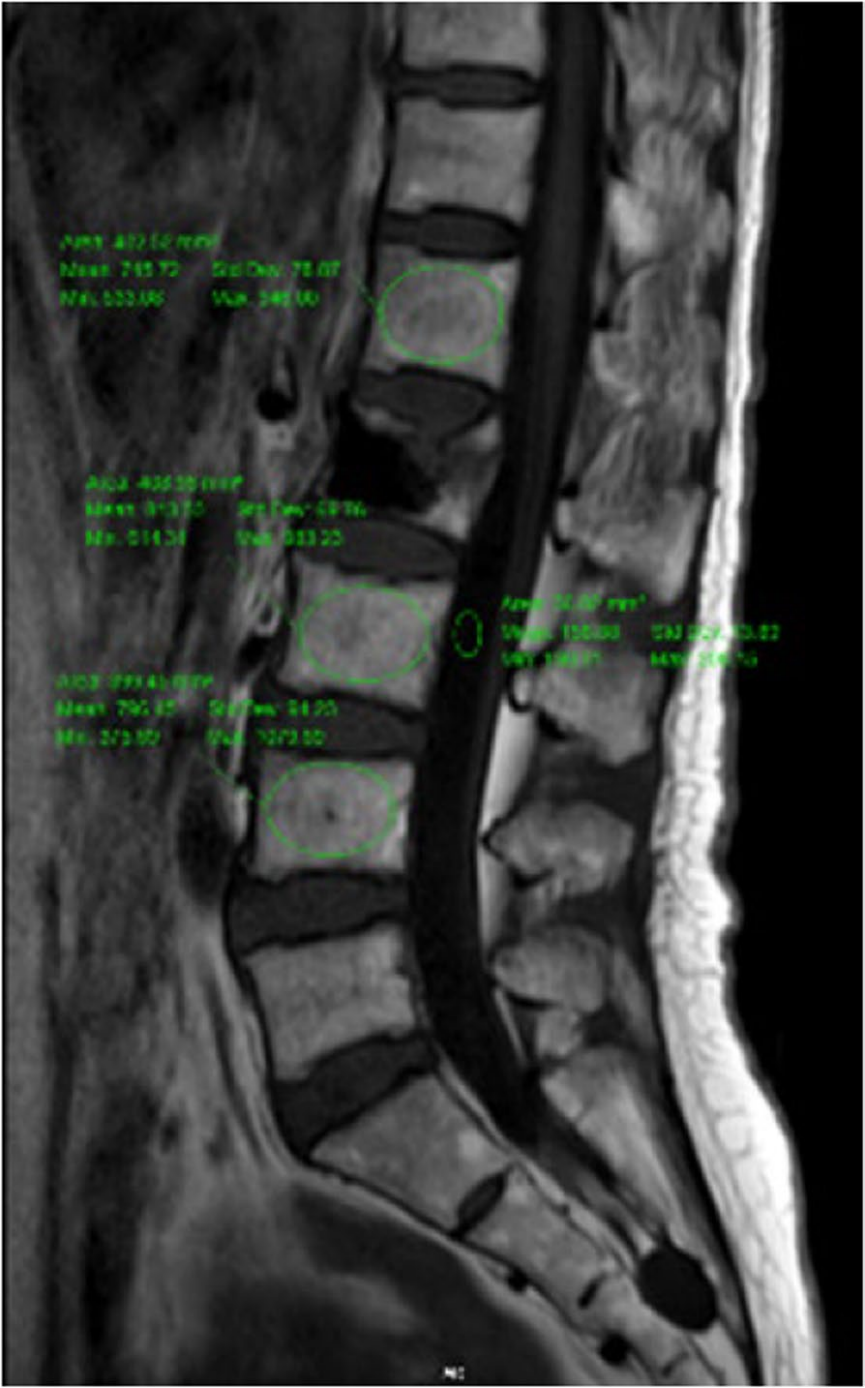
Non-contrast-enhanced T1-weighted MRI image shows the SI of L1, L3, L4 and SI of CSF using ROIs
